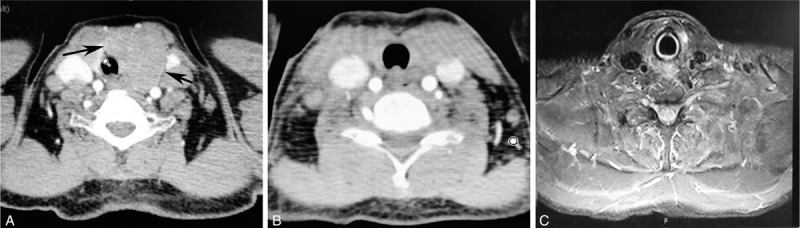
Computed tomography (CT) scans of the neck. CT picture before subtotal thyroidectomy showed diffuse thyroid enlargement in the left lobe compressing the trachea (A, black arrow). CT picture after subtotal thyroidectomy (B) and the most recent MRI picture (C) revealed no local recurrence.
